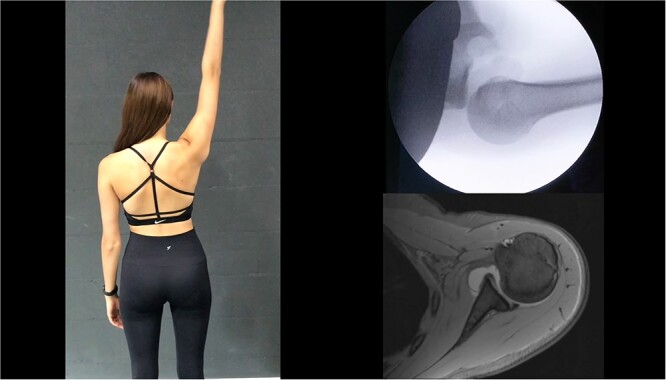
Patient with functional posterior shoulder instability (FPSI). The clinical image shows dynamic posterior subluxation of the humeral head during every elevation of the right arm, which can be visualized under fluoroscopic imaging. Despite this severe instability, no structural defects are visible on magnetic resonance imaging.
